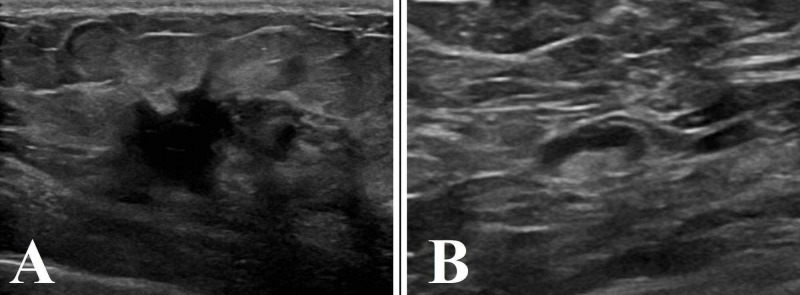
Breast ultrasound A: left breast showing a 2.6 x 1.4 x 1.9 cm irregular mass with speculated margins; B: ipsilateral axilla showing one abnormal appearing LN with thickened cortex measuring 4 mm LN: lymph node
